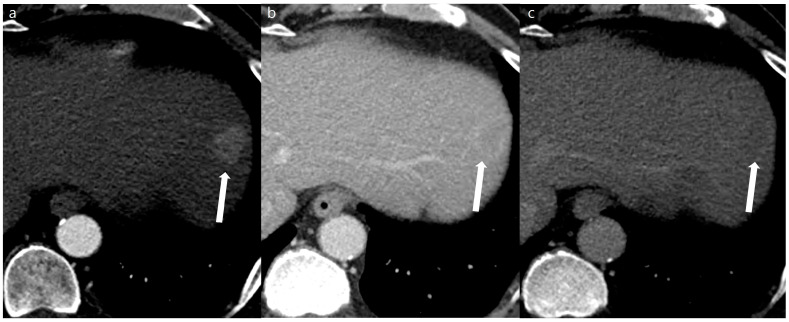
A 53-year-old man with a 2 cm sized hepatocellular carcinoma at segment 2 of the liver (arrows). Arterial phase (a), portal phase (b), and delayed phase (c) images of a standard-dose CT using hybrid iterative reconstruction show a well-enhancing nodule in the arterial phase (a), with washout on the portal phase (b) and the delayed phase (c).
